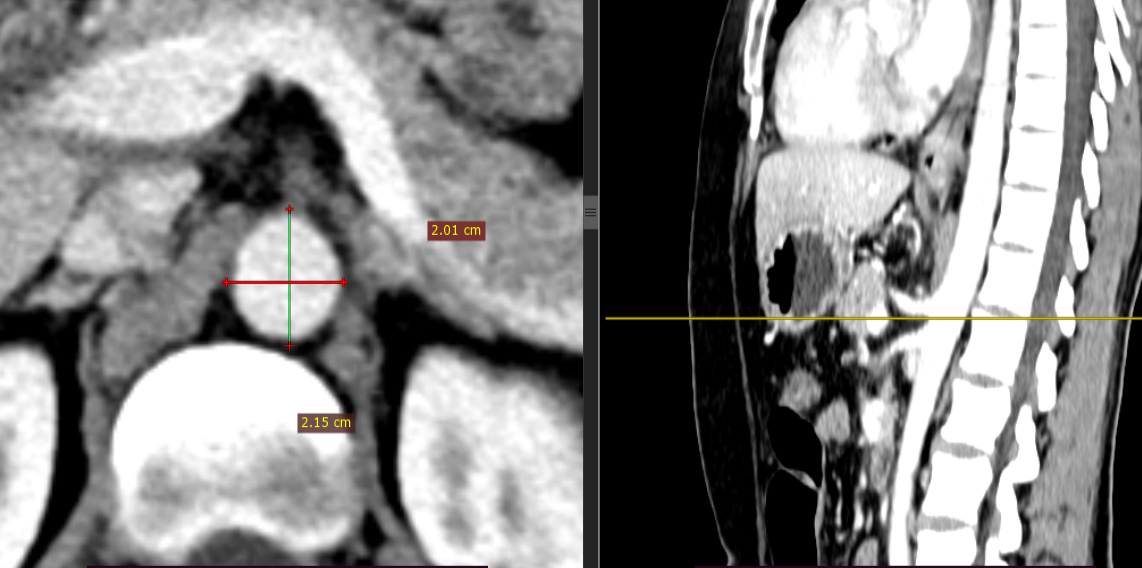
Axial image taken at T12 level (Aortic hiatus level) showing outer to outer CT measurement
